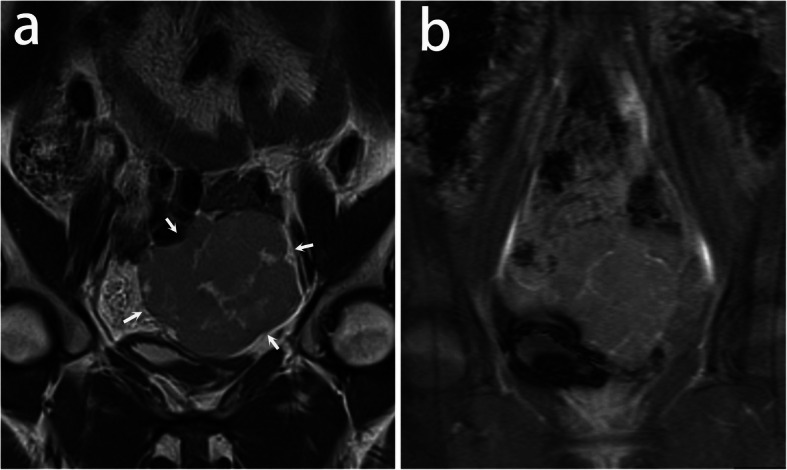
A 24-year-old woman with dysgerminoma in the left ovary. a Coronal T2-weighted image demonstrates a solid mass (arrows) with multiple hyperintense septa because of edema. b Coronal T1- weighted contrast enhanced image shows the mass has a moderate enhancement. The septa are enhanced linearly and markedly
